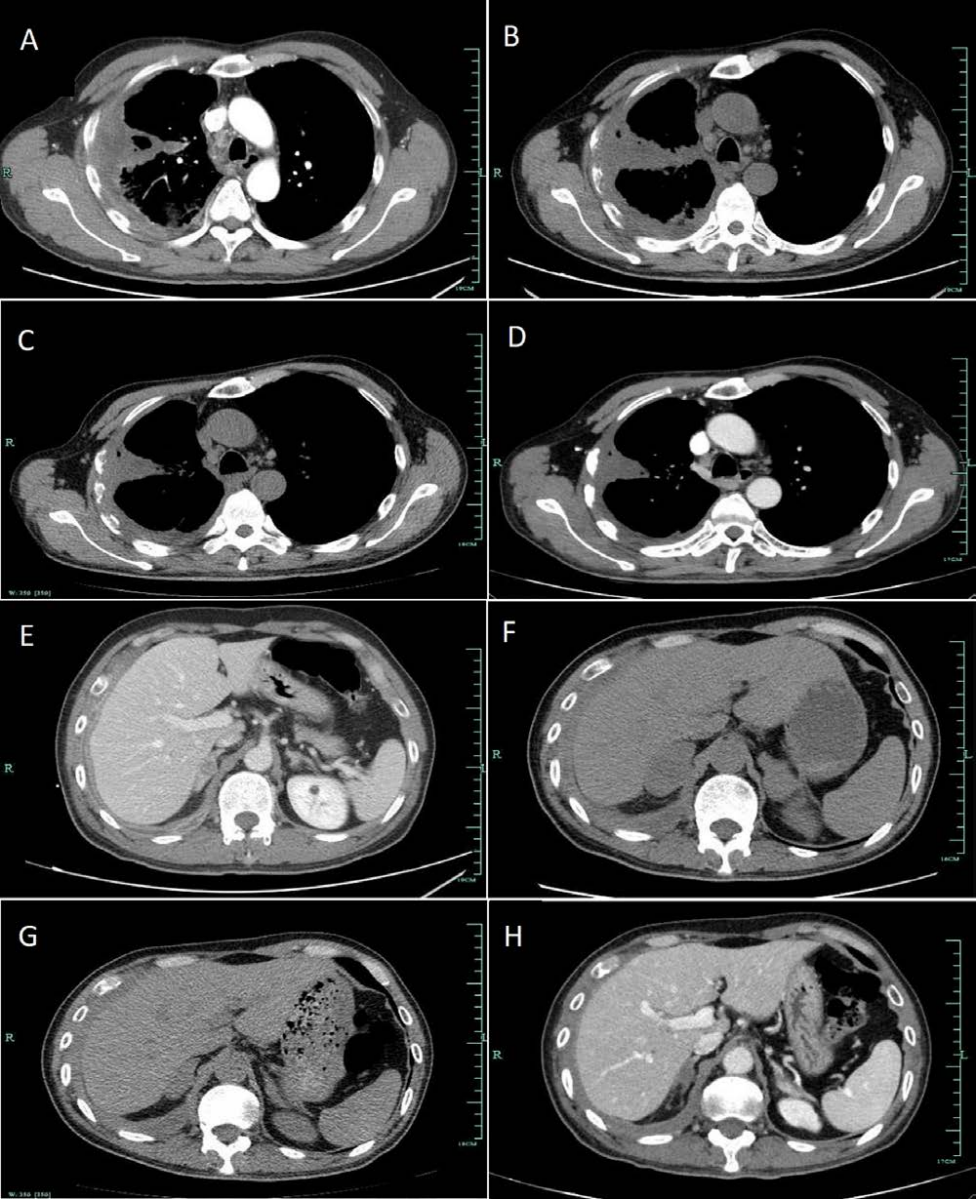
Changes in lesions of the lung (A-D) and bilateral adrenal glands (E-H) on CT during treatment with EGFR-TKIs and pembrolizumab monotherapy. A/E: pretreatment. B/F: EGFR-TKI targeted therapy for 2 months. C/G: Pembrolizumab monotherapy for 1 cycle. D/H: Pembrolizumab monotherapy for 5 cycles.
